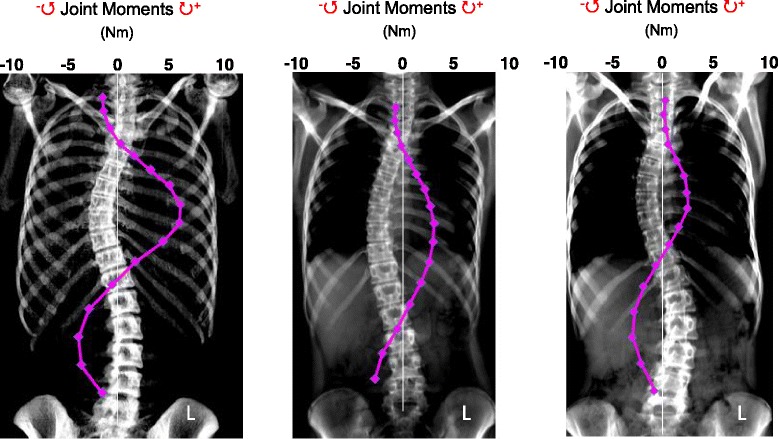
Examples of coronal CT images (from Patients 4, 8 and 10) showing the variability of joint moment distribution for three patients (Anterior-Posterior view). The scale shows joint moments in Nm and + ve is a clockwise moment
